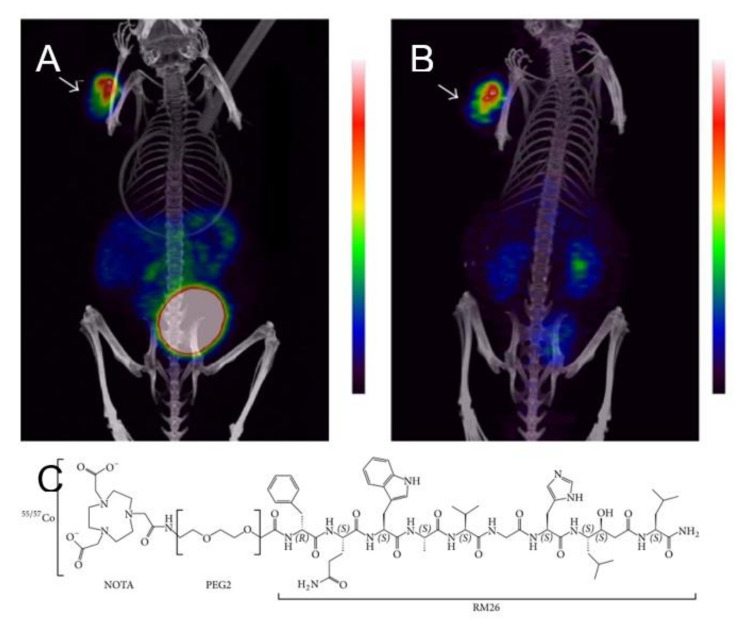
PET/CT images of [^55^Co]Co-NOTA-PEG_2_-RM26 (C) at 3 h PI (A) and 24 h PI (B) show the reduction in background signal between early and late timepoint imaging. [13].
